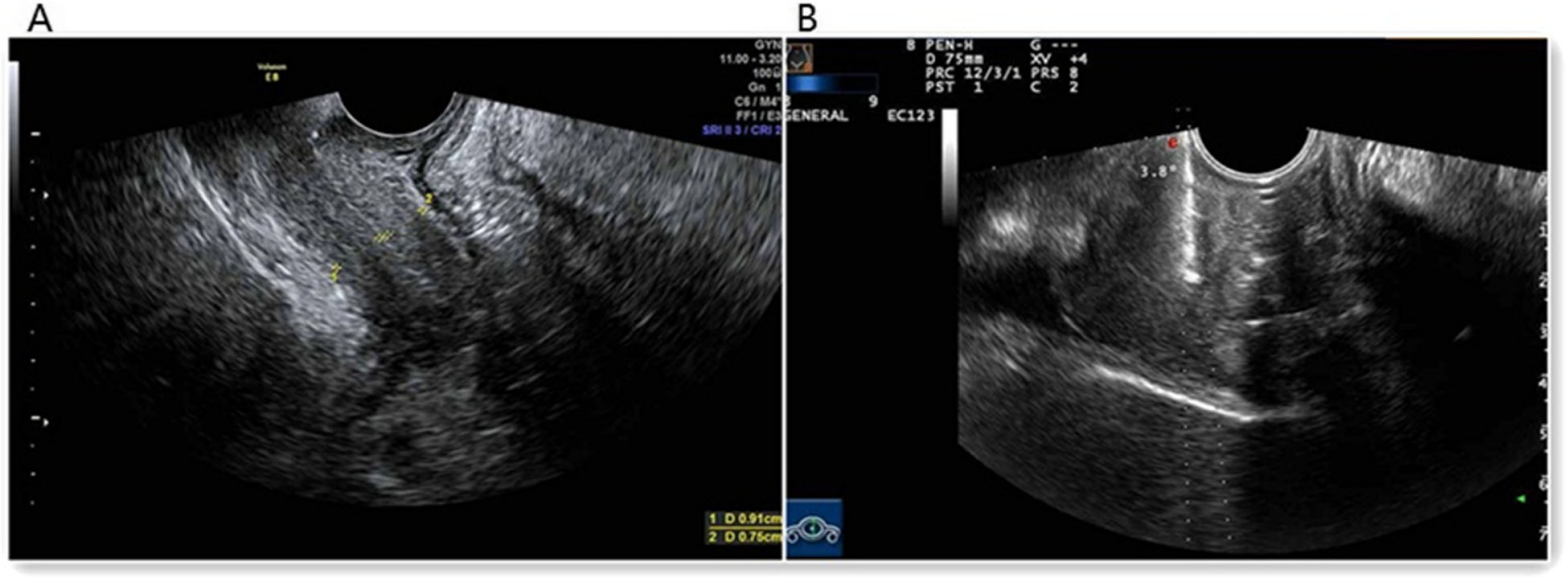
Ultrasound examination image. a.: TVS showed clear uniform thickening of the vaginal wall. b: TVS-guided vaginal wall biopsy
